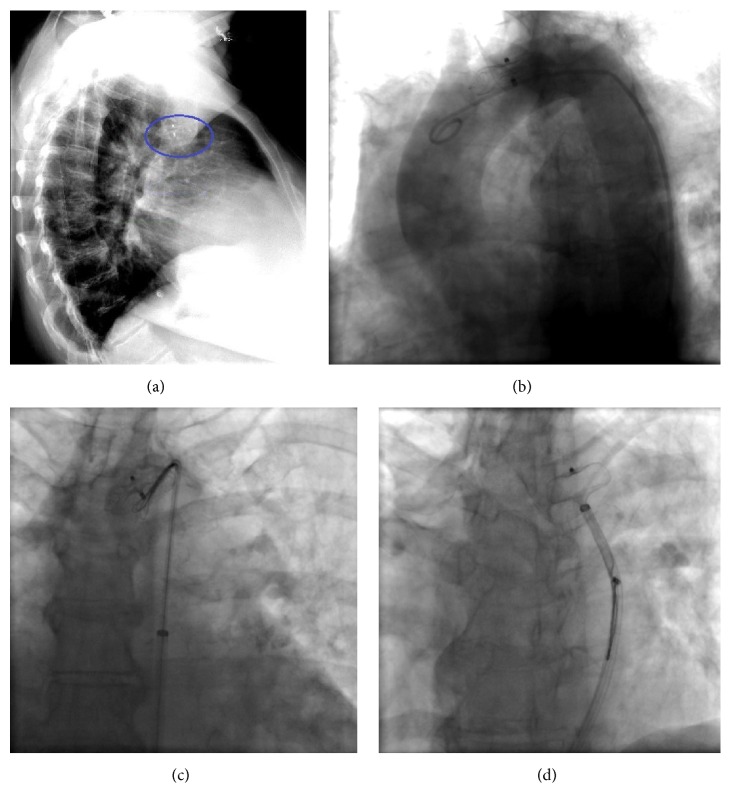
(a) Chest radiograph, lateral view showing the embolized ASO. (b) Aortic arch angiography using angled pigtail catheter. (c) Using En-Snare, capturing of the metallic tip of the right atrial disc of the ASO. (d) Retraction of the entire ASO into the long cook sheath.
